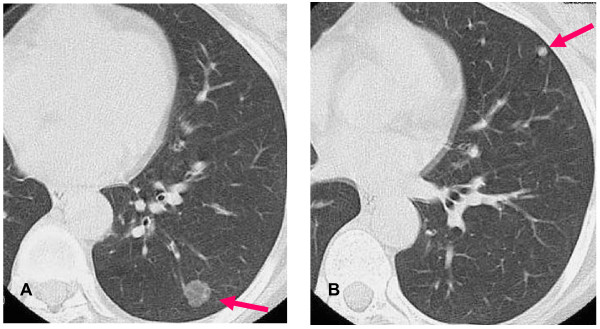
CT scan of our patient's chest on admission to our hospital. (A) Chest CT scan shows GGO (arrow) in her left S10 lung segment of 1.3 cm in size. (B) Chest CT scan shows a solitary small nodule (arrow) of 5 mm in diameter in her left S4 segment.
